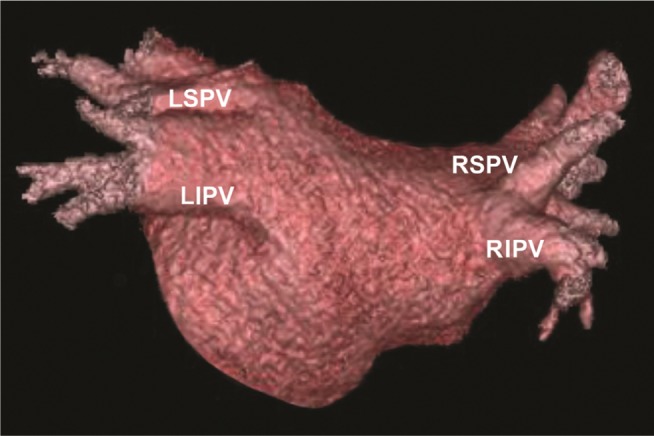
Computed tomographic reconstruction of the left atrium, posterior view.
